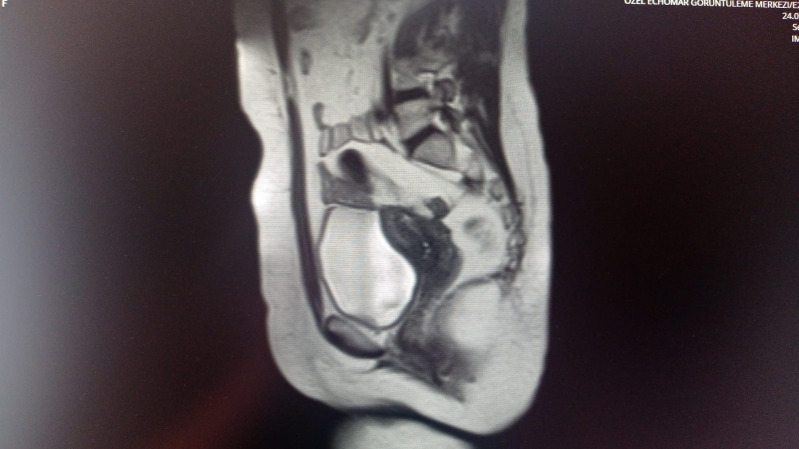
Postoperative magnetic resonance image of a patient who underwent laparoscopic adenomyomectomy resulting in a huge fundoposterior myometrial defect precluding pregnancy.
